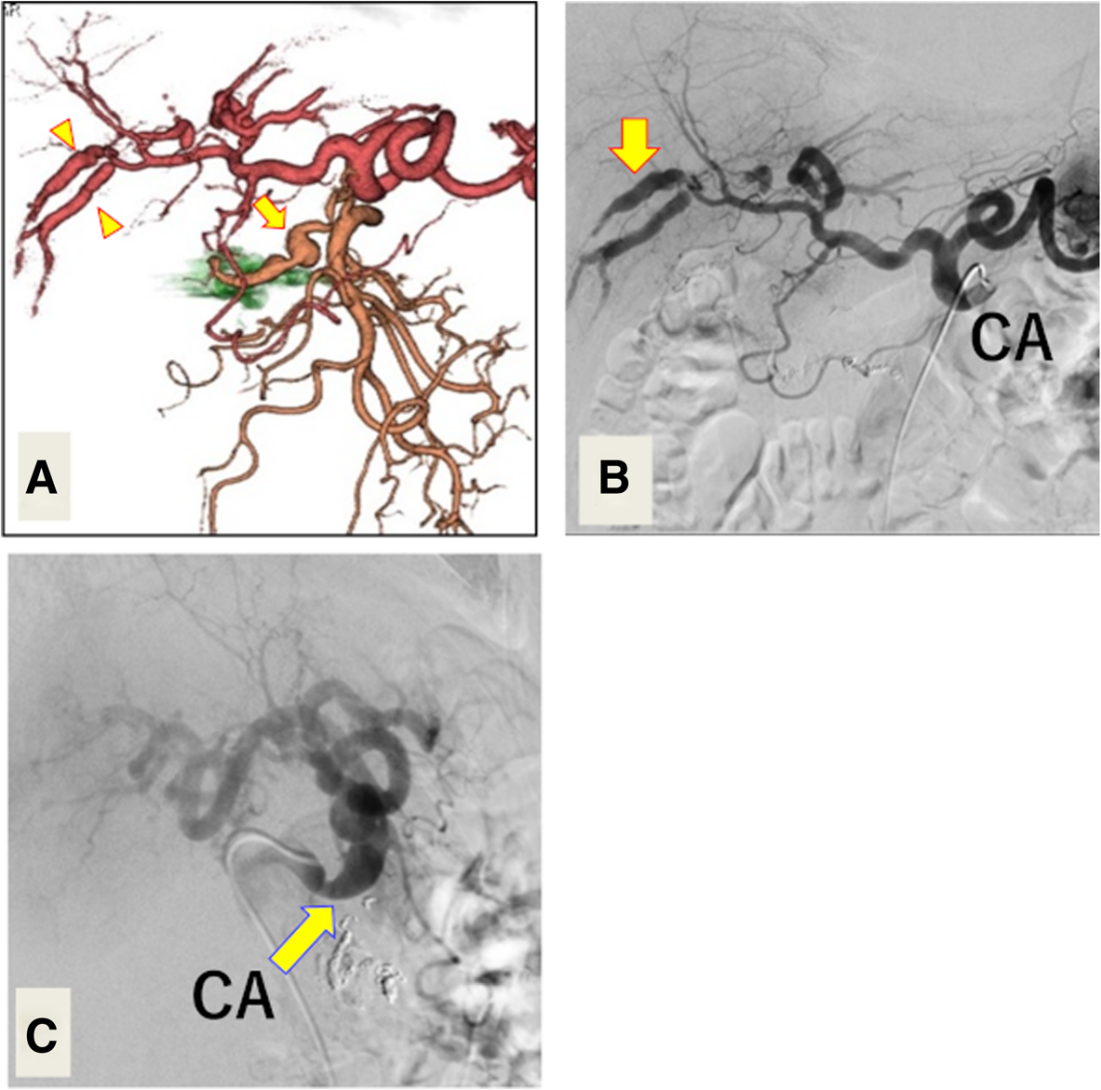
Enhanced computed tomography and diagnostic angiography performed prior to the second transcatheter arterial embolization. a Multiplanar reformatted image of the follow-up enhanced computed tomography on day 21 shows transverse pancreatic artery aneurysm (arrow) and hepatic artery aneurysms (arrowheads). b Diagnostic angiography on day 24 demonstrates sustained blood flow in the CA hepatic artery aneurysms (arrow). c Sagittal view of the angiography demonstrates severe stenosis of the CA (arrow). CA, celiac artery
